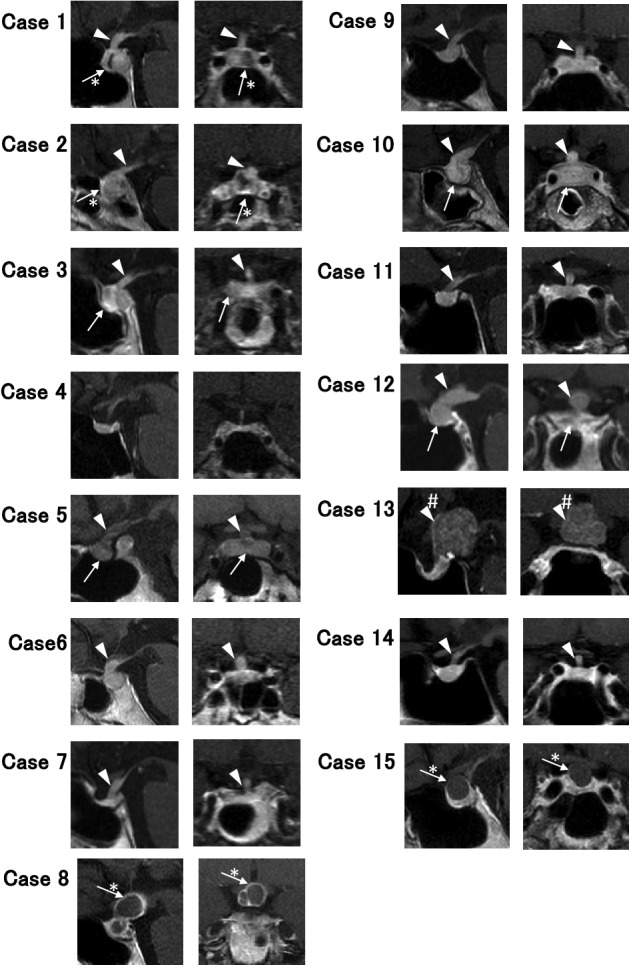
T1-weighted MR images of the pituitary in the 15 patients. The images from Case 5 were not enhanced because the patient was pregnant. All other images were enhanced by gadolinium contrast medium. Sagittal (left) and the coronal (right) sections from each patient are shown. Arrowheads indicate thickened pituitary stalks, and arrows indicate enlarged pituitary glands. *Indicates a cystic component, and #Indicates a well-defined tumor.
